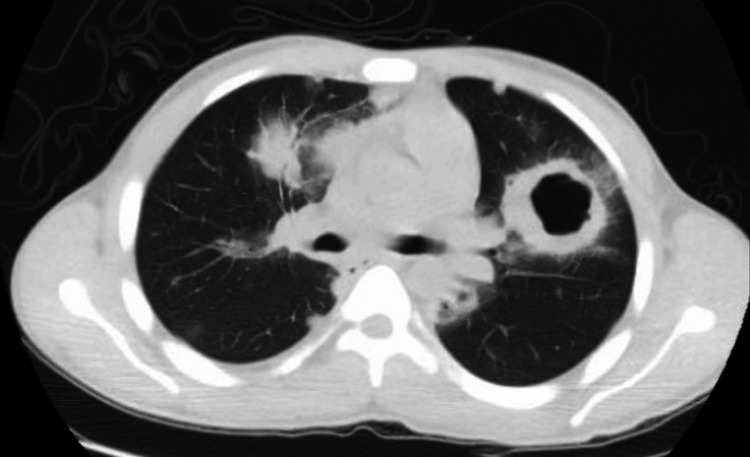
Axial CT chest demonstrating cavitary pulmonary nodules consistent with granulomatosis with polyangiitis at initial diagnosis.
